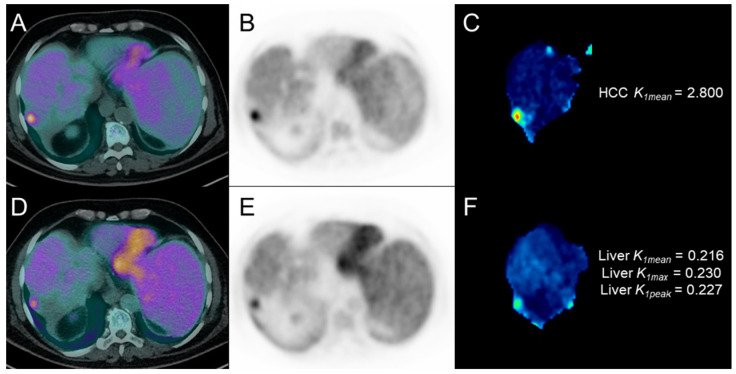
^13^N-ammonia PET images of patient #12, with an HCC lesion of the 7th liver segment, at baseline ((A): transaxial PET/CT; (B): transaxial PET; (C): *K_1_* parametric map of the HCC lesion) and at post-treatment ((D): transaxial PET/CT; (E): transaxial PET; (F): *K_1_* parametric map of non-neoplastic liver parenchyma).
